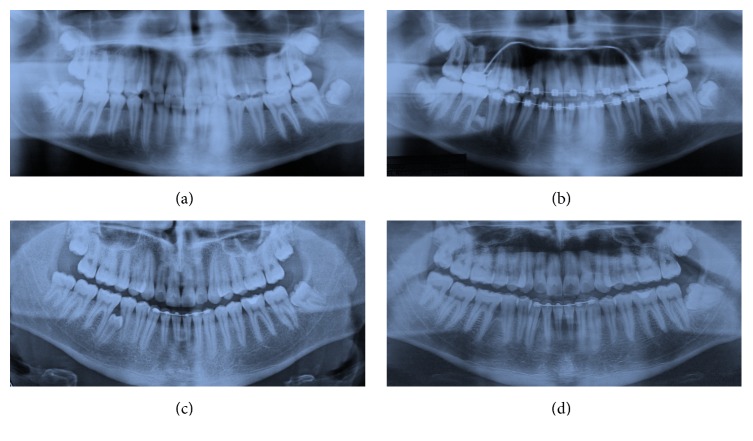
Case 1 radiography: before treatment 2004 (a), before rebonding, ST in 4.5–4.6 region, 2006 (b), 5 years after debonding 2011 (c), and after the extraction of the ST 2013 (d).
